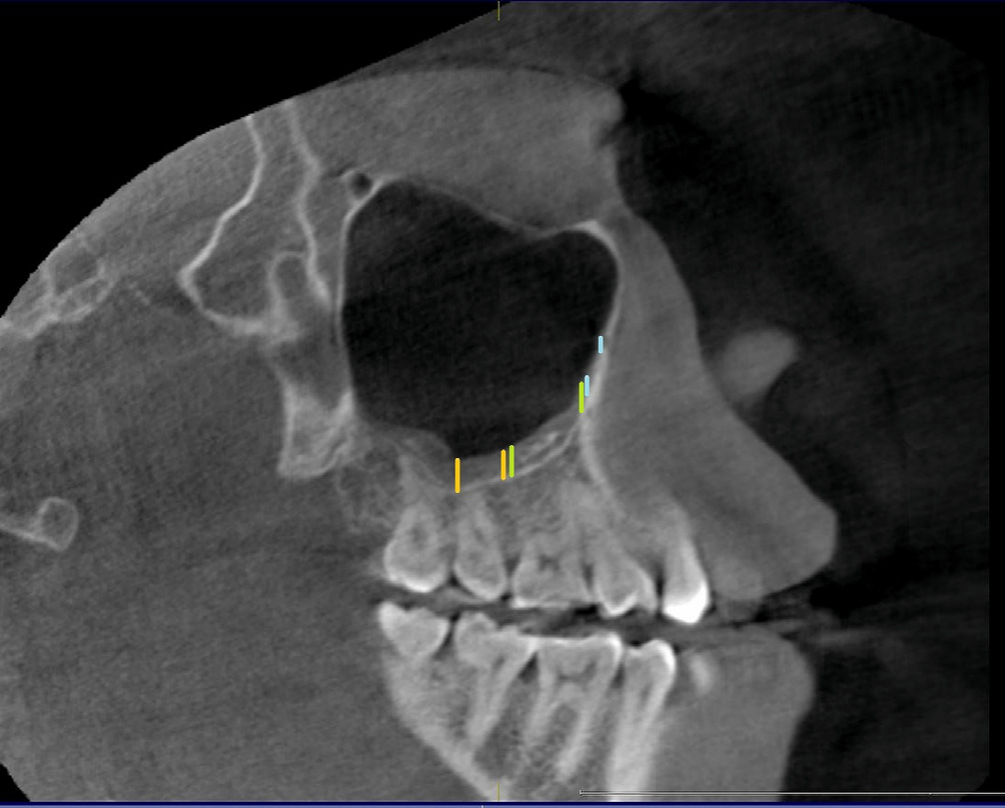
Sagittal CBCT section showing the six sites at which the mucosal thickening was measured in dentate study subjects. The mesial and distal sides of the second.
